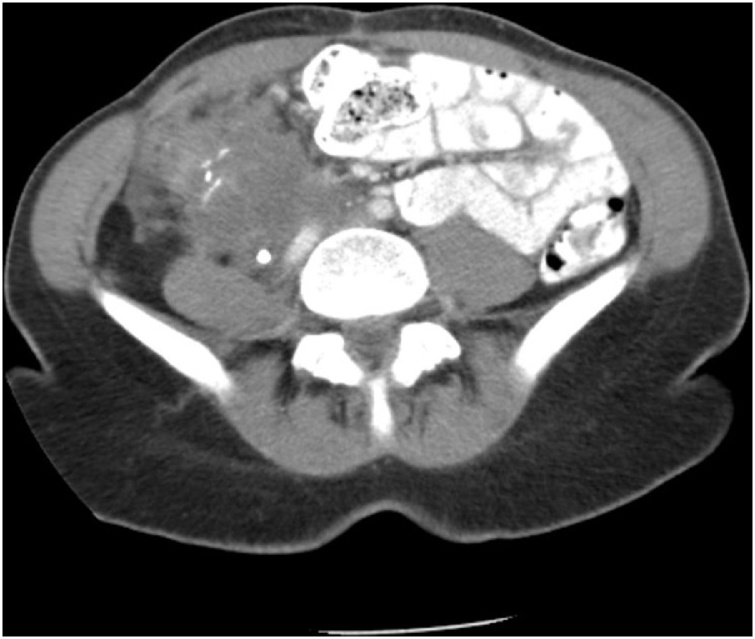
CT cut through the mid-abdomen in a 39 year old woman with recurrent right colon cancer. The staple line from the prior ileocolic anastomosis is in the central portion of the mass. A stent is in the obstructed right ureter.
